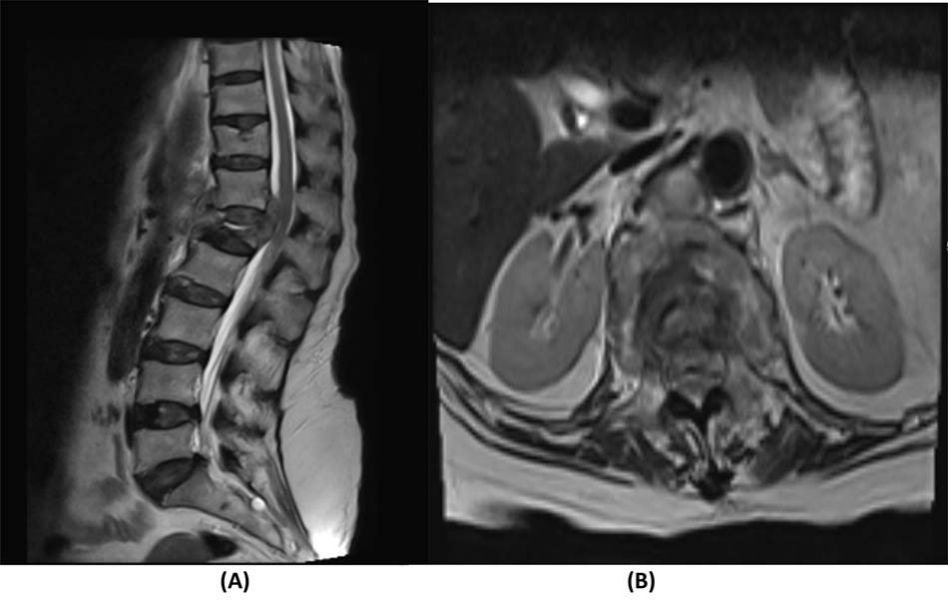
(A) T2-weighted sagittal lumbar spine MRI demonstrating a pathological fracture of L1 with spinal canal and neural foramina stenosis and cord edema and epidural and prevertebral soft tissue components. (B) T2-weighted axial lumbar spine MRI of the corresponding level of L1 vertebral body.
